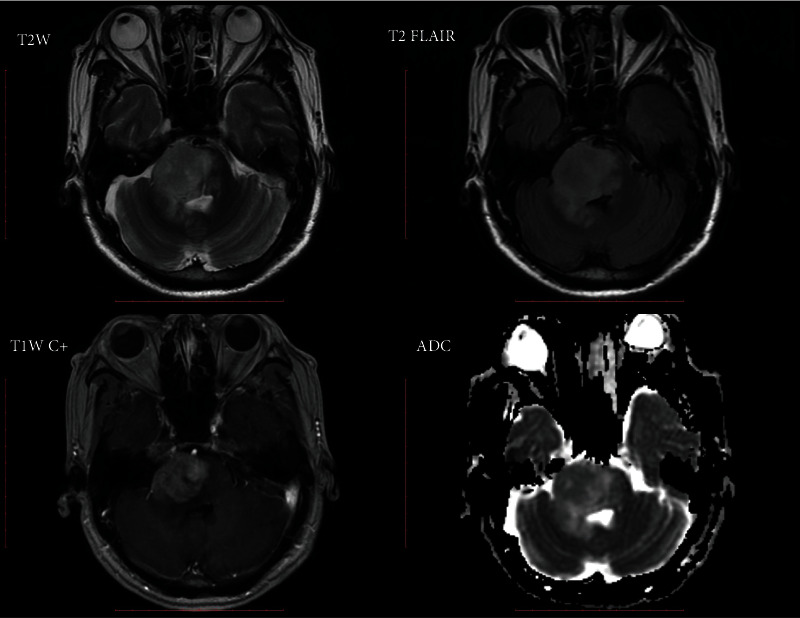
A 65-year-old man with an IDH1-wildtype glioma (diffuse astrocytoma, WHO grade II). The tumor is located in the brainstem with marked contrast enhancement, deep white matter invasion, a necrosis proportion of <33%, and a mixed tumor behavior (T1<FLAIR).
